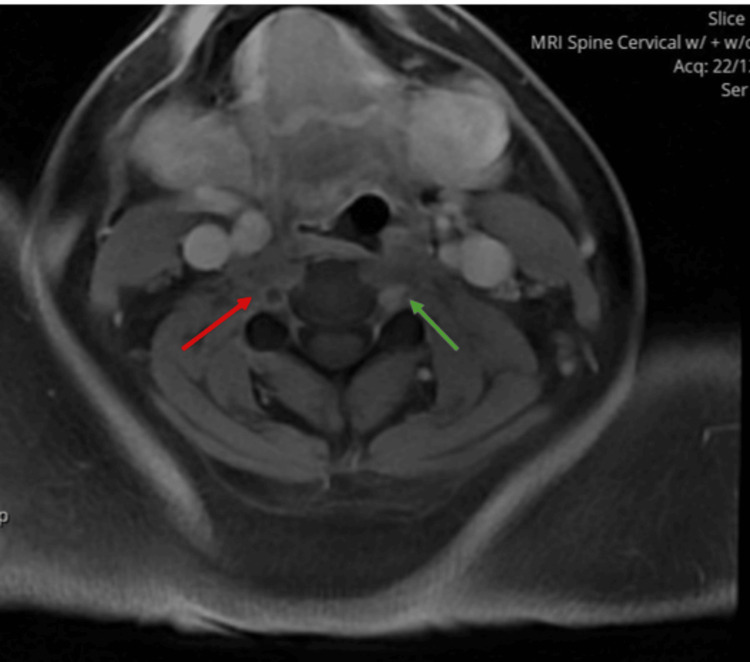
Filling defect in the right vertebral artery on MRI Magnetic resonance imaging (MRI) of the neck shows a filling defect within the right vertebral artery (red arrow), suggestive of thrombus formation or progression of dissection, in contrast to the normal enhancement of the left vertebral artery (green arrow).
